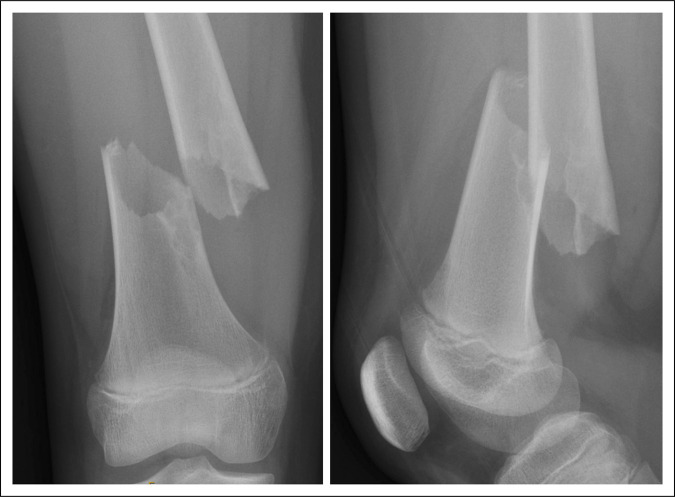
Preoperative radiographs of a displaced distal third femoral shaft fracture through a nonossifying fibroma in a skeletally immature patient.
